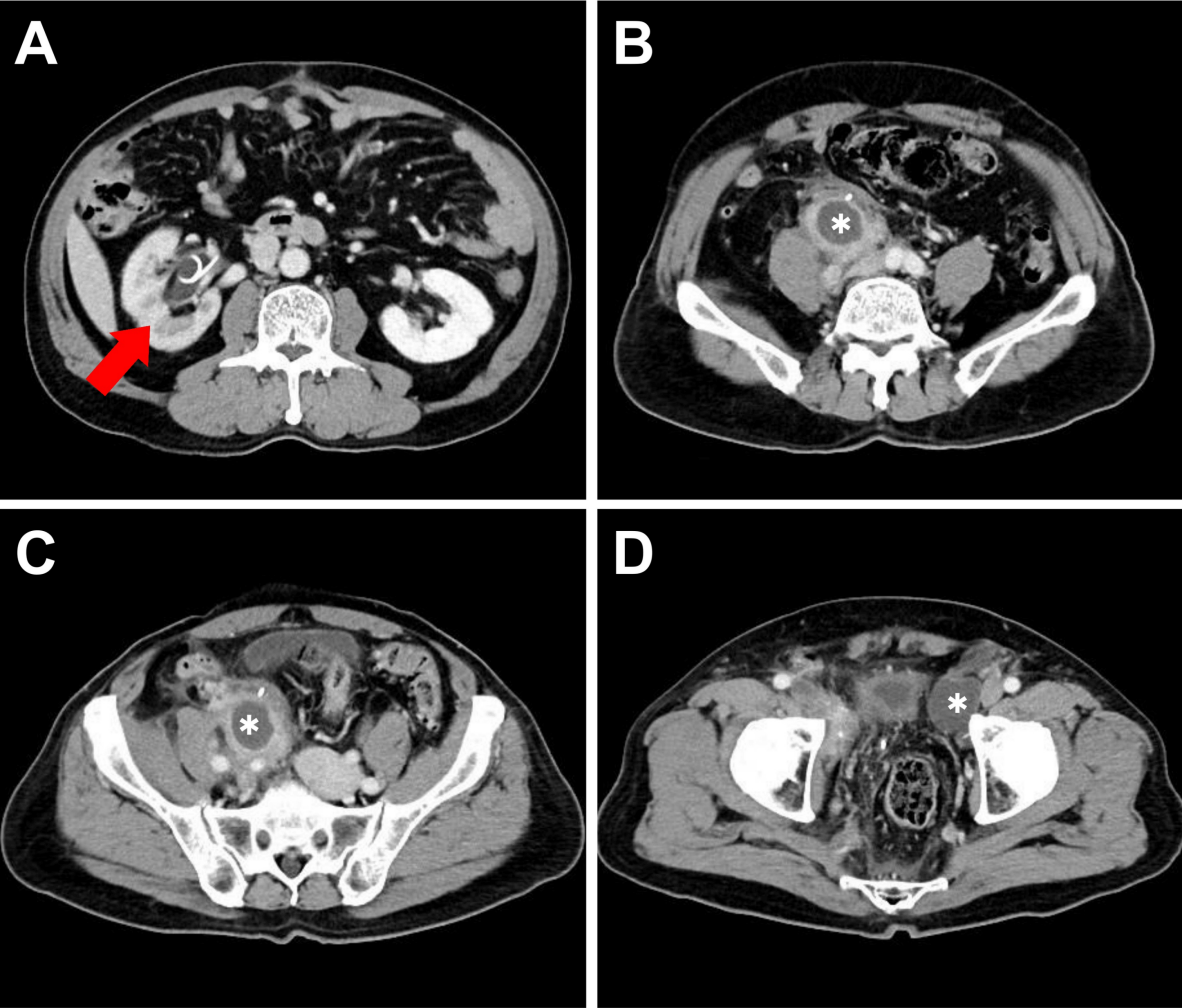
Three-month follow-up contrast-enhanced CT images in axial view after right ureteral stent placement (A) Right hydronephrosis has improved (red arrow). (B-D) The lymphoceles (white asterisks) have partially regressed but become loculated. CT: computed tomography
